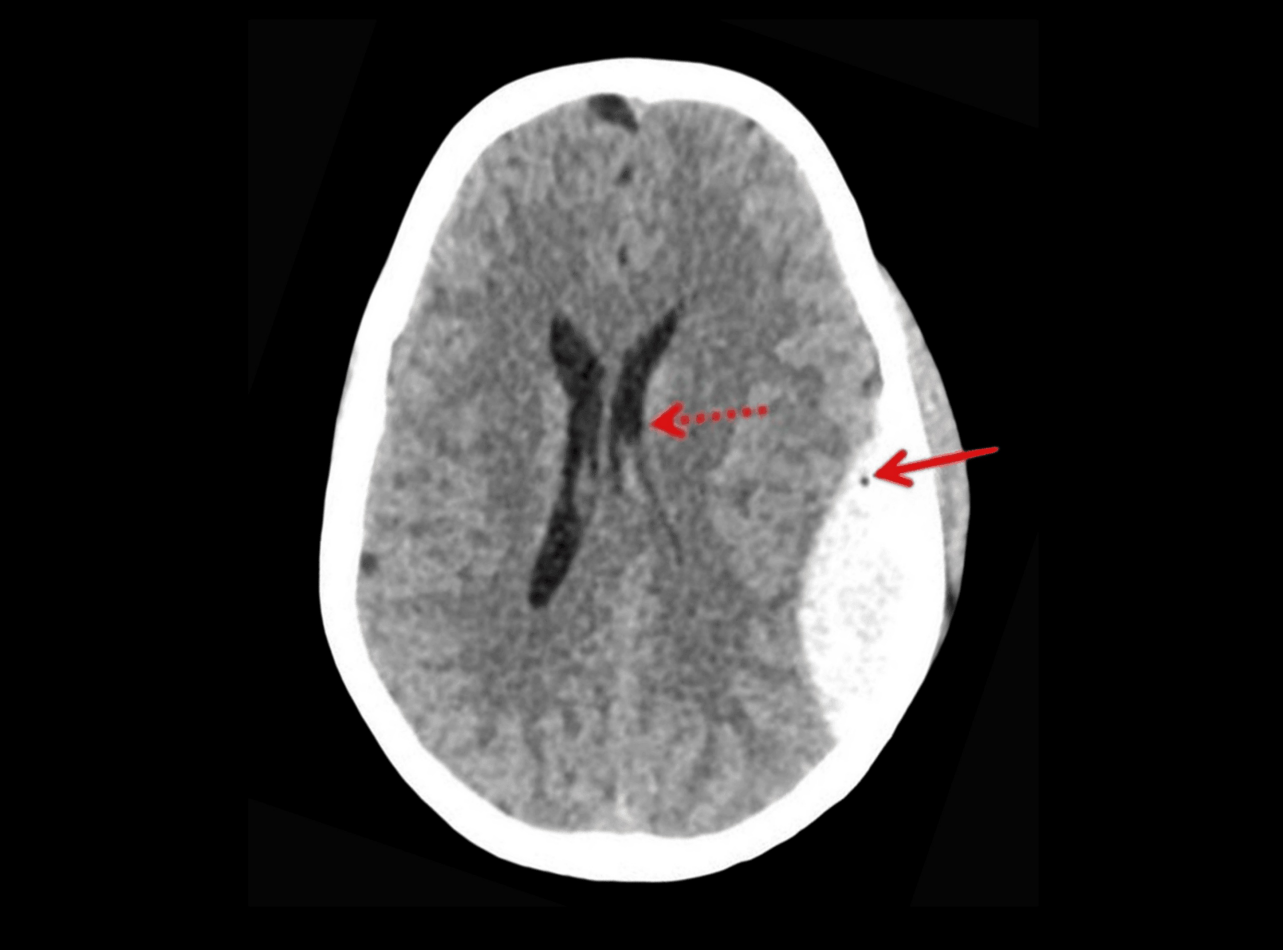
Preoperative NCCT showing left occipitoparietal EDH with air foci within (arrow) and causing compression of the ipsilateral lateral ventricle (dotted arrow). NCCT: non-contrast computed tomography; EDH: extradural hematoma
